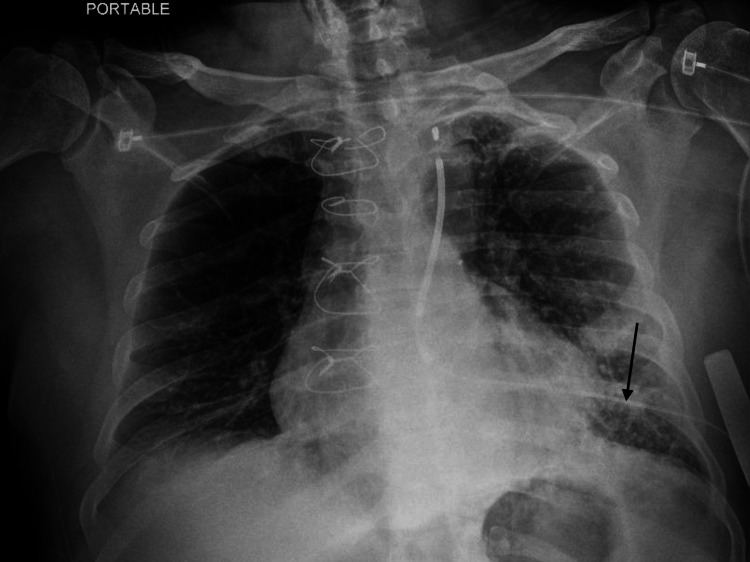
Chest radiograph depicting patchy left lower lung infiltrates (arrow)
